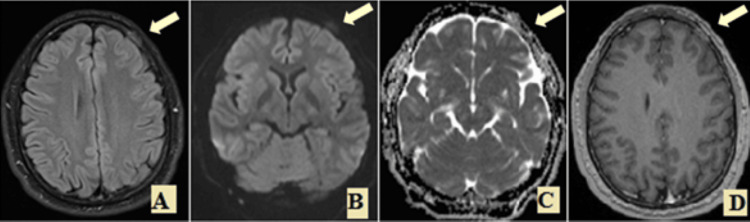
Axial T2/FLAIR, axial DWI/ADC Map (B,C), and axial gadolinium enhanced T1(D): a follow-up three months later, shows diminution of the intra-/extra-osseous soft tissue process and decrease of the meningeal enhancement as well as the contrast enhancement and the diffusion restriction (arrow). DWI: Diffusion-weighted imaging, ADC: Apparent diffusion coefficient
